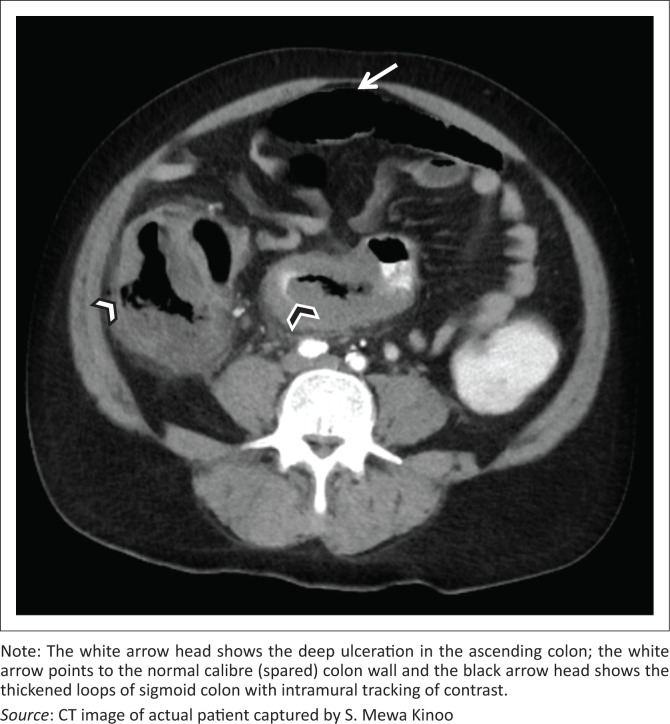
Computed tomography scan with triple contrast.
